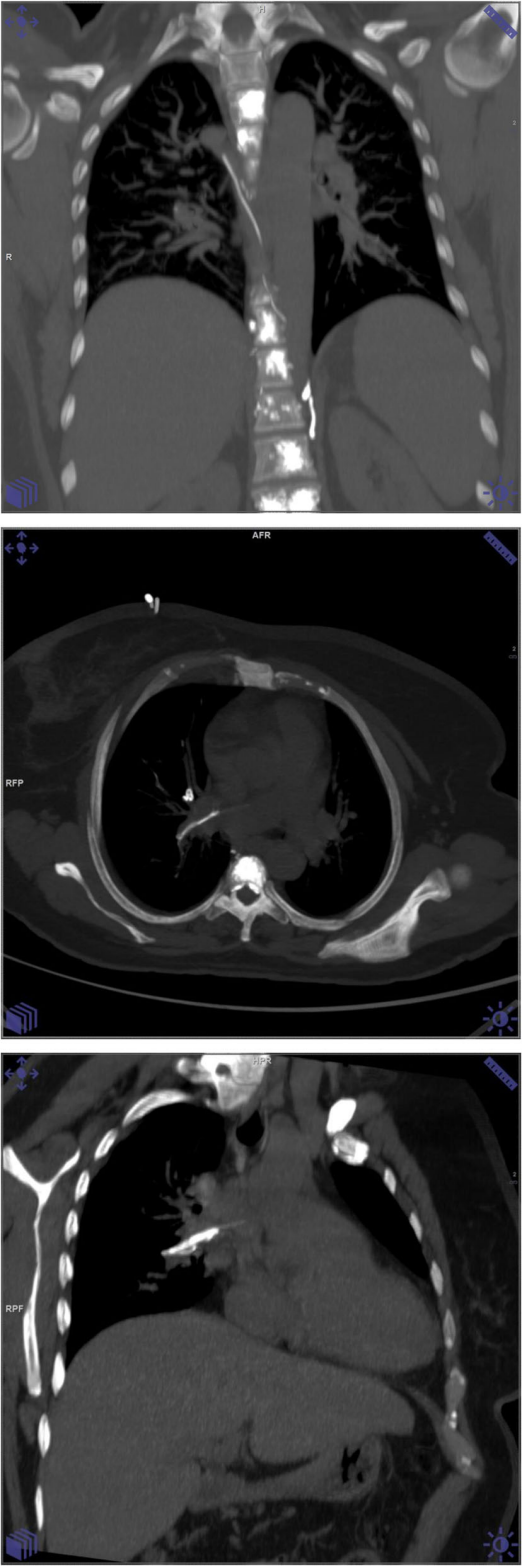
Selected coronal image with maximum intensity projection of unenhanced chest CT showing multilevel vertebral augmentation by cement. There is a branching linear density along the course of the lumber veins and the azygus/hemiazygus system representing cement leak/ intravasation. Axial and coronal oblique MIP images are showing a similar linear density in the right main pulmonary artery extending to the right superior segmental branch of the right lower lobe representing cement pulmonary emboli
